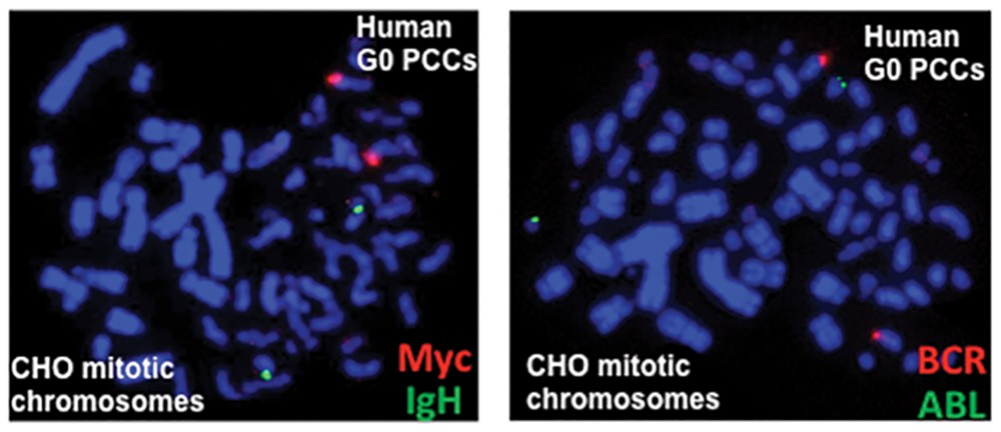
Detection of gene loci in G0 PCCs by FISH using gene specific probe sets. Fluorescently labeled gene probe sets (c-Myc-Texas Red and IgH- Fluorescein; BCR-Fluorescein and ABL-Texas Red) were used for detection. PCCs prepared from 3 Gy X-rays treated lymphocytes were used for detection.
